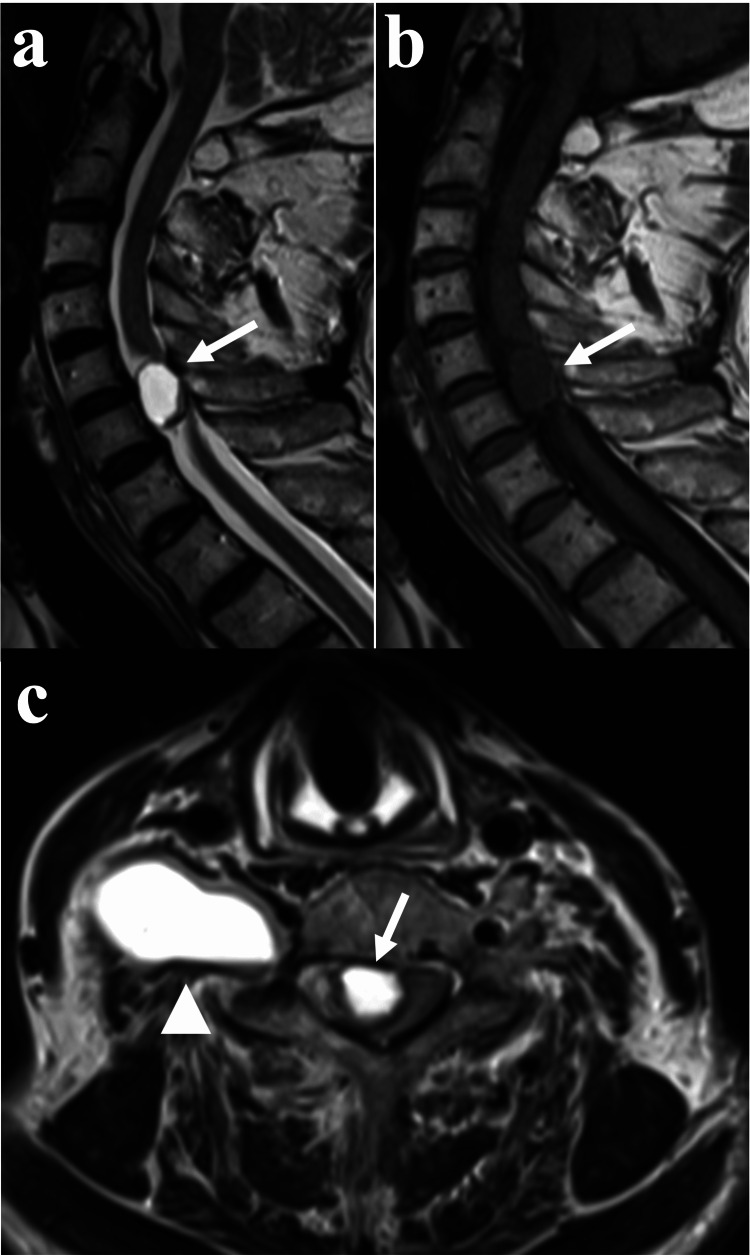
MRI of the cervical spine (a) Sagittal T2-weighted image showing a hyperintense area at the C5-6 level. The arrow indicates the hypointense lesion attached to the tumor. (b) Sagittal T1-weighted image showing an isointense area. The arrow indicates the isointense lesion attached to the tumor, indicating a hematoma. (c) Axial T2-weighted image showing a hyperintense lesion severely compressing the spinal cord and a hypointense to isointense lesion (arrow). The hyperintense lesion is spread anteriorly through the foramen and anterior part including fluid-fluid level (arrow head), indicating hemorrhage.
